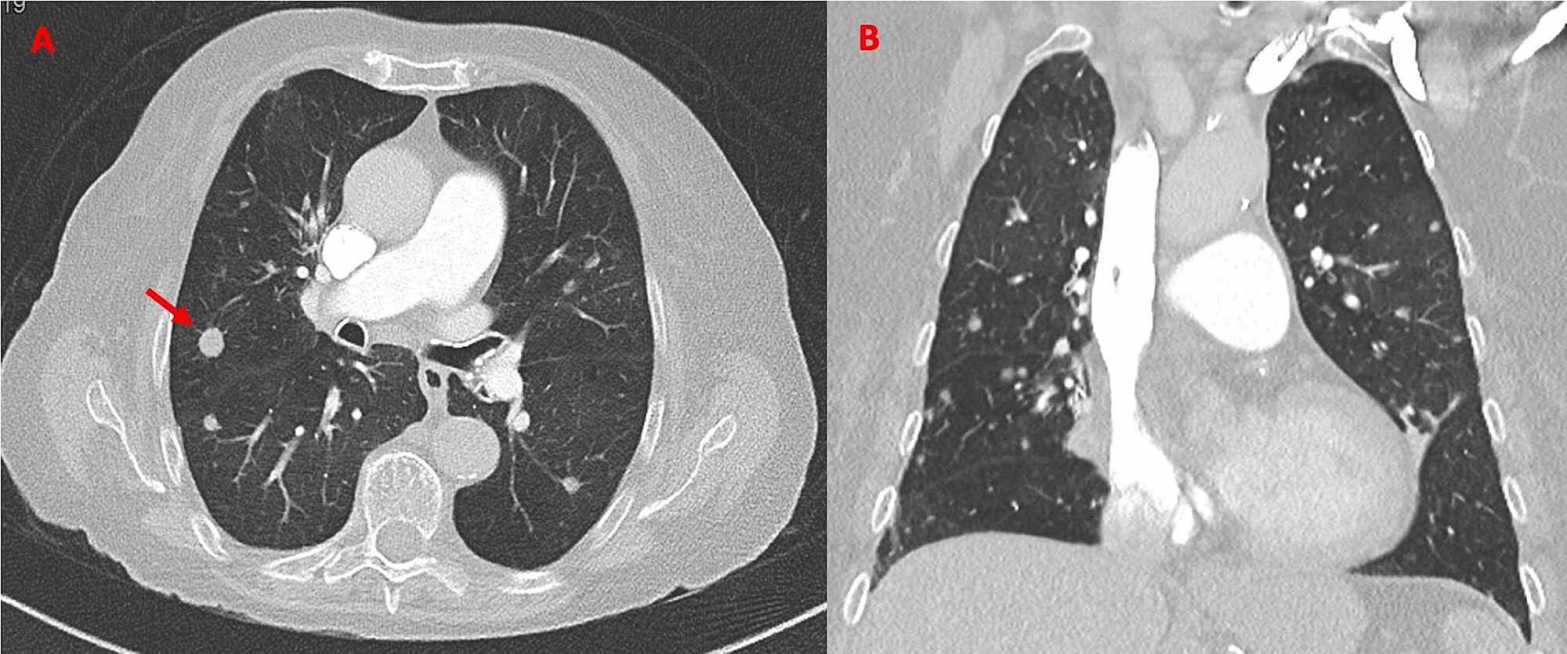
Chest CT angiography from 2019 (admission) showing nodules in the lung parenchyma, with the largest located in the right superior lobe, with the biggest diameter of 9 mm (arrow). Panel A: transversal plane; Panel B: coronal plane. CT, computed tomography
